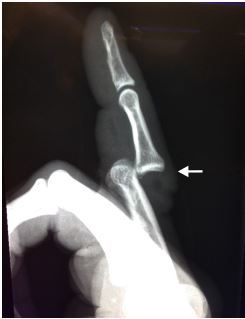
Lateral radiograph of right index finger demonstrating dorsal dislocation of the proximal interphalangeal joint without evidence of fracture (arrow).
